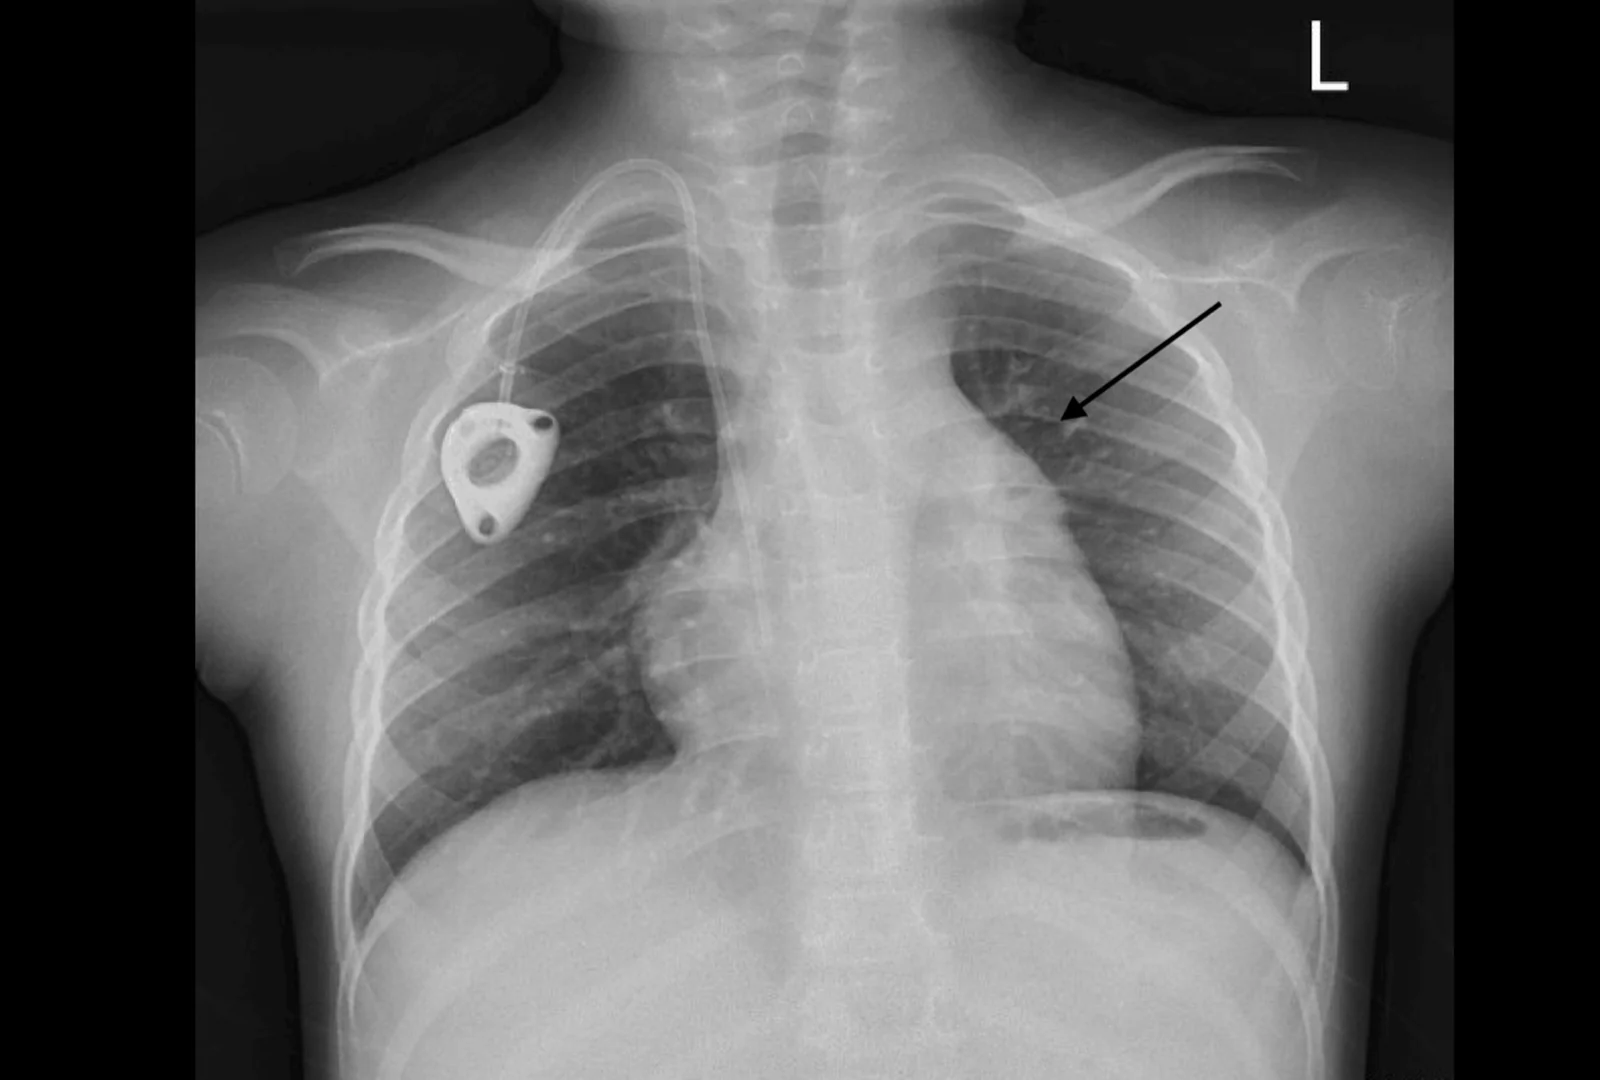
Frontal radiograph of chest Showing near-resolution of mediastinal mass. A mild bulge in the mediastinum, as shown by the black arrow, is present.
